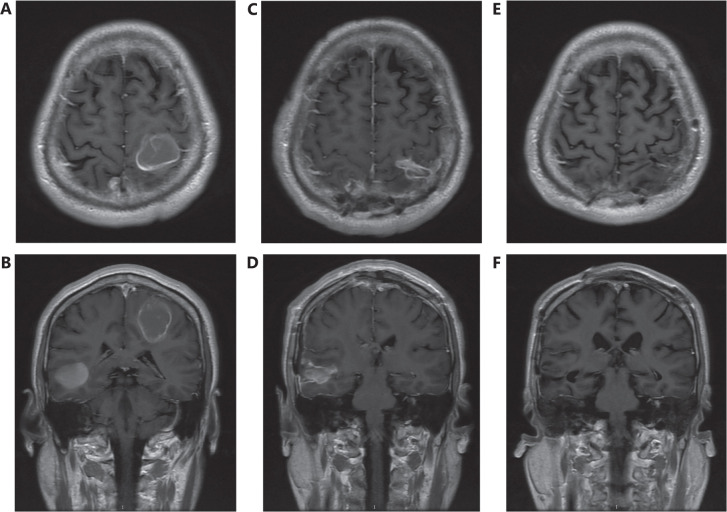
Preoperative axial (A) and coronal (B) T1 MRI with contrast, demonstrating 3 cerebral metastases: 1 in the left parietal lobe, 1 in the right parietal lobe, and 1 in the right temporal lobe. One month postoperative axial (C) and coronal (D) MRI showing total resection of all 3 metastatic lesions. One year postoperative axial (E) and coronal (F) MRI showing no tumor recurrence.
